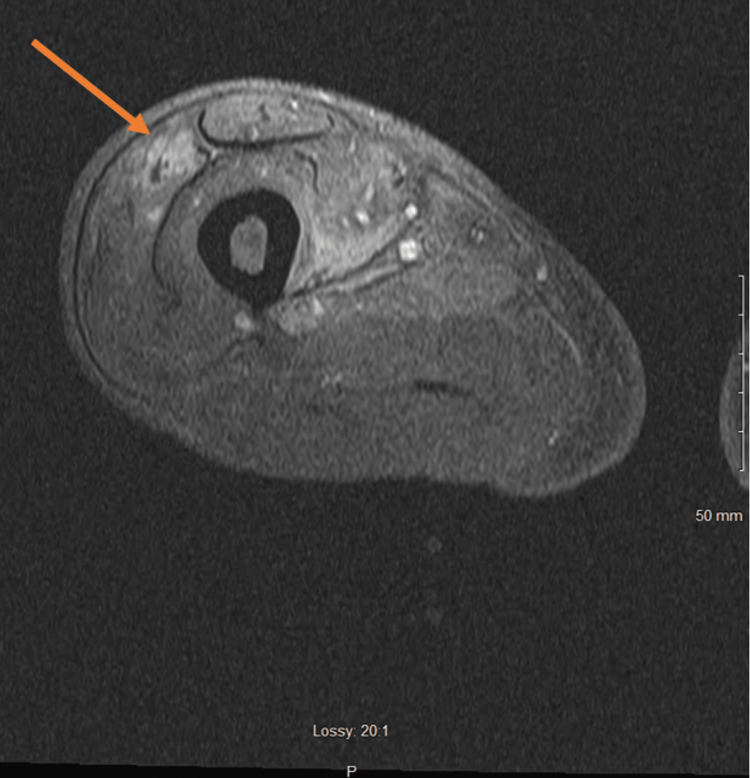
Magnetic resonance imaging of the right femur with and without contrast T-2 weighted MRI highlighting muscle edema and muscle enhancement involving the anterior compartment and the abductor muscles, suspicious for myositis. There are irregular areas of non-enhancement within the vastus lateralis and medialis muscles which may represent myonecrosis or developing abscess.
